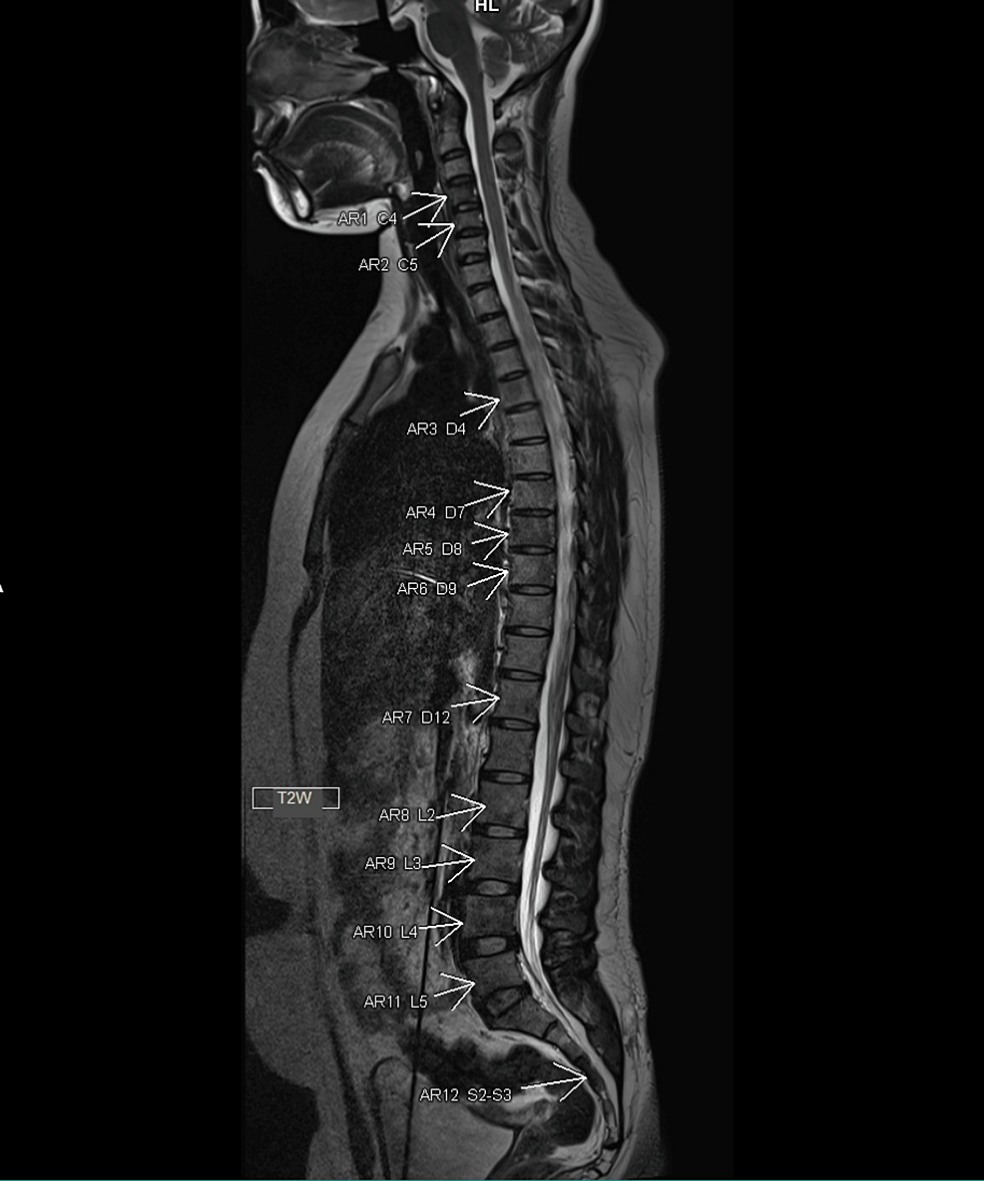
MRI scan. Multiple sclerotic lesions (indicated by arrows) in the multiple levels of vertebral bodies (level was indicated in image) like C4, C5, D4, D7, D8, D9, D12, L2 to L5, and S2 to S3. These lesions appear hypointense, which is unusual for unicentric Castleman’s Disease.
